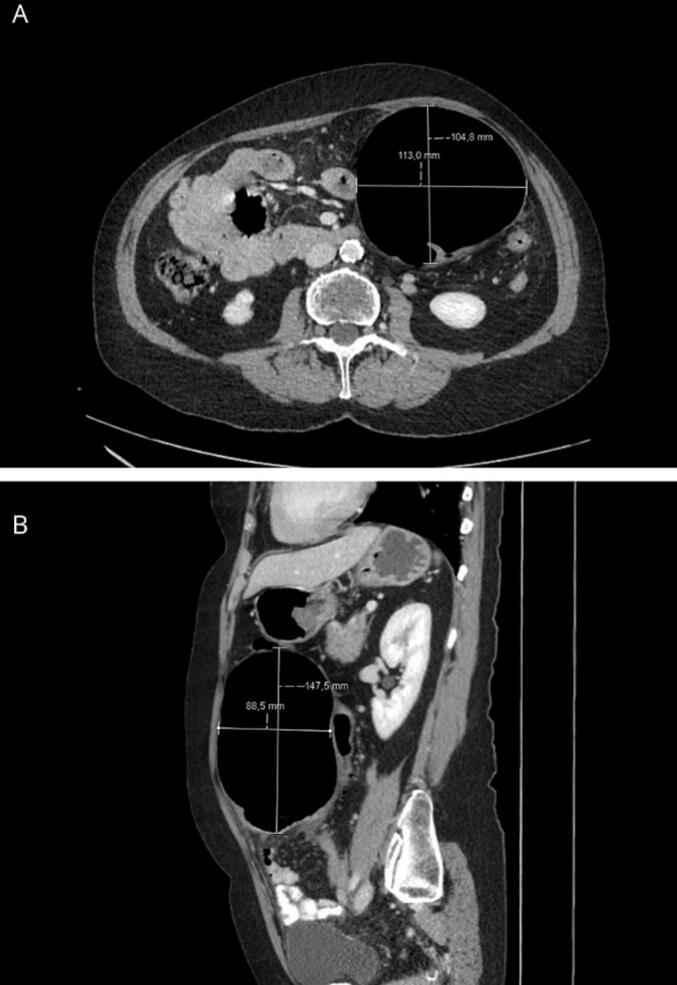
CT scan demonstrating a large, air-filled cyst adjacent to the sigmoid colon (Balloon sign). (A) Axial view, (B) Sagittal view.
